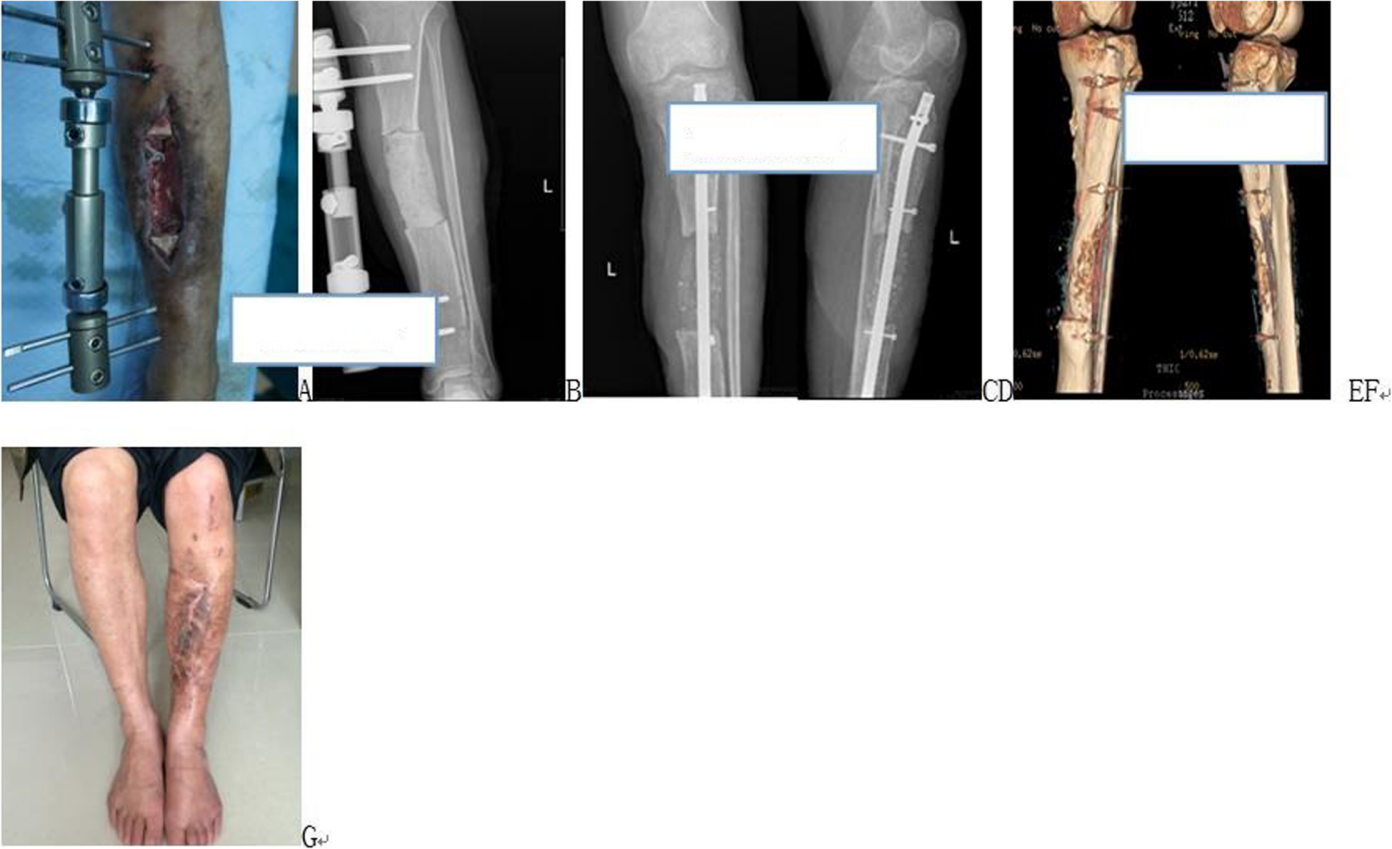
A patient with an infective left tibial bone defects who was treated with induced membrane cancellous bone graft. a Preoperative appearance. b Postoperative X-ray films after skin flap repair and bone cement packing. c and d Postoperative X-ray films 3 months postsurgery. e and f CT three-dimensional reconstruction to evaluate bone healing 1 y postsurgery. g Appearance 1 year postsurgery
